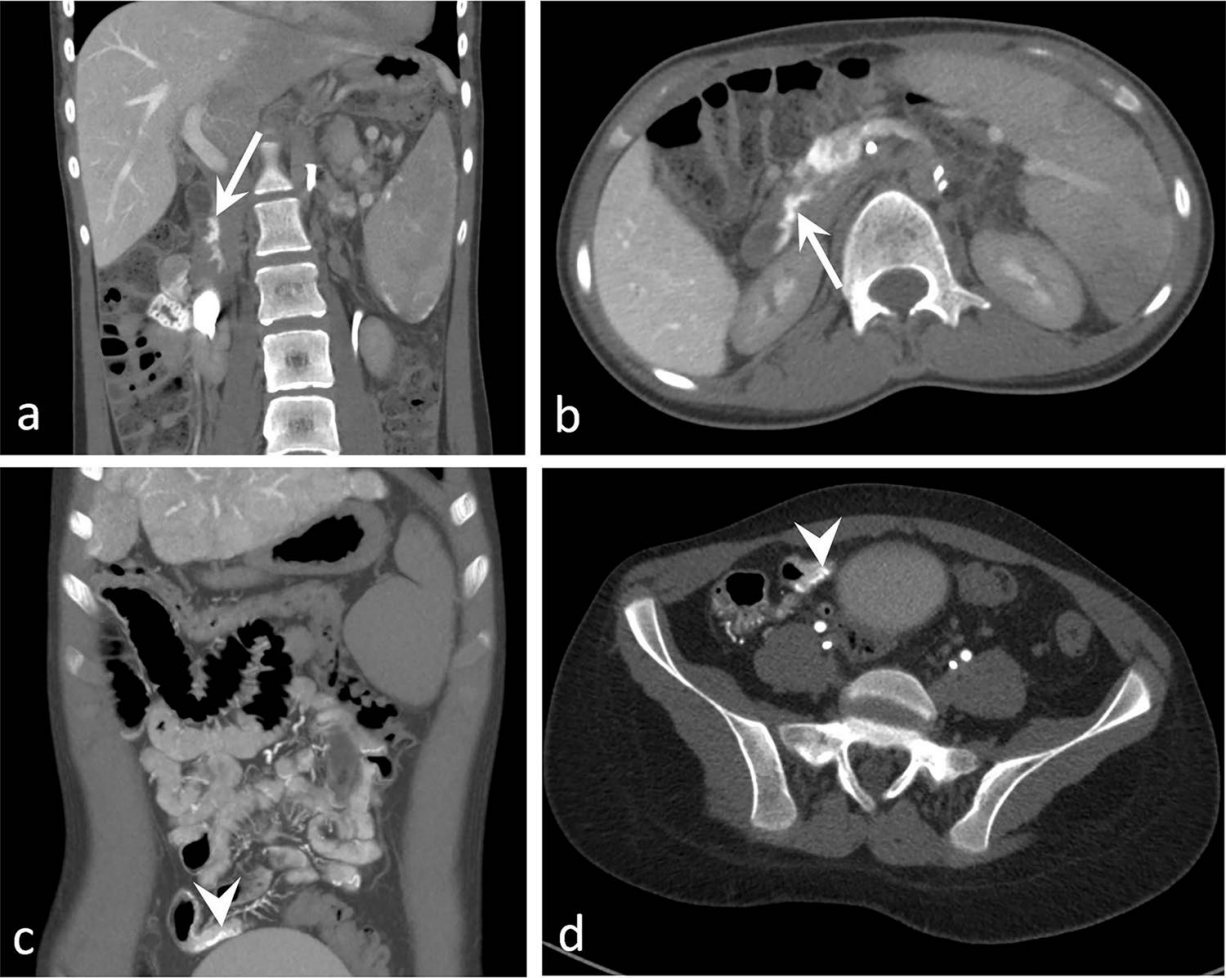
Axial and coronal images of CT-AP of two 16-year-old male patients with congenital hepatic fibrosis (a, b) and hepatoportal sclerosis (c, d). CT-AP detected small duodenal varices not described in UEG (arrow) and small intestinal varices (arrowheads) not detectable in CE-CT.
